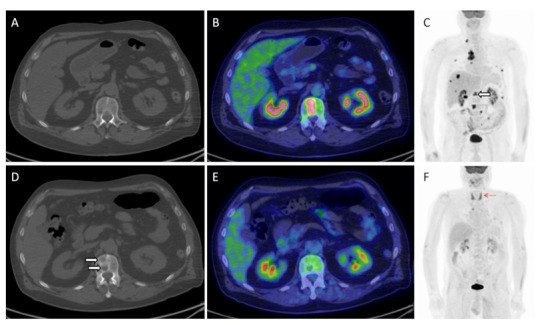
Herein, the imaging findings of a 66-year old male with metastatic NSCLC investigated before (A–C) and after 3 cycles of pembrolizumab (D–F). An overall response to treatment is easily visible on MIP (maximal intensity projection) images (C,F), including a complete metabolic remission of all bony lesions ((C); white hollowed arrow). On the contrary, morphological imaging proved the appearance of a new bone lesion in the first lumbar vertebra ((A,D); white arrows), which in fact corresponded to a healed metastasis on PET/CT (B,E). Note also the appearance of diffuse thyroid uptake ((F); red arrow), consistent with thyroiditis, one of the irAEs that typically predicts treatment response and good patient’s outcome.
